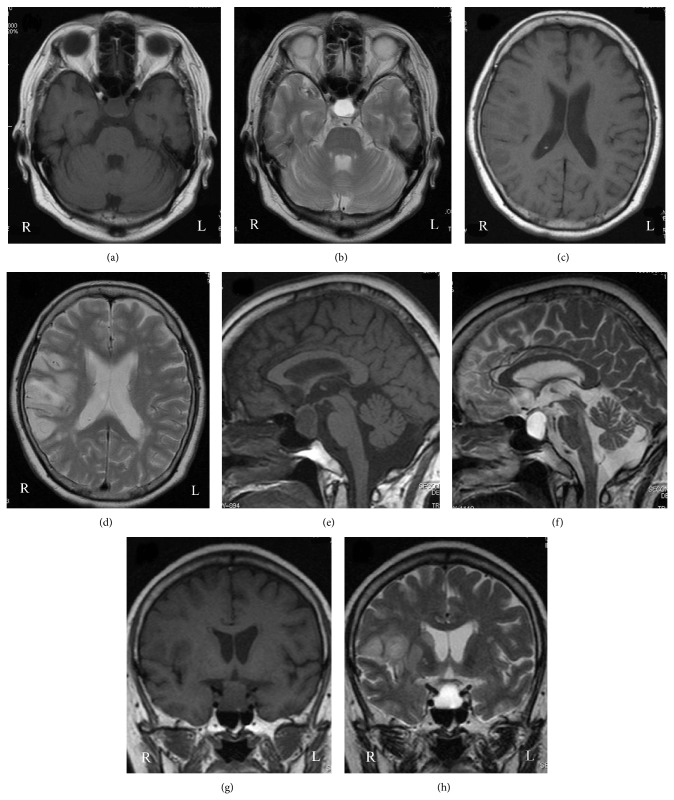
Axial, sagittal, and coronal views of the MRI showed isointensity on T1WI (a, e, and g) and high intensity on T2WI (b, f, and h) in the suprasellar region. Axial and coronal views of the MRI presented isolow intensity on T1WI (c, g) and high intensity on T2WI (d, h) in the territory of the right middle cerebral artery.
